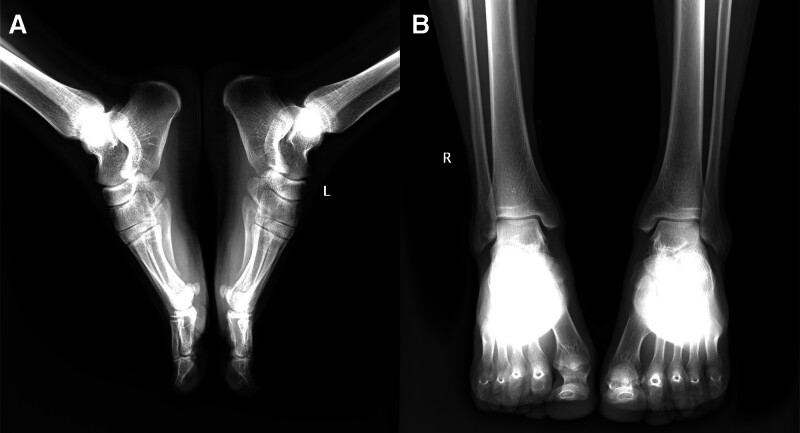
Preoperative ankle X-rays showing no fracture, dislocation, or other abnormalities. (A) Lateral view; (B) Anteroposterior view.
